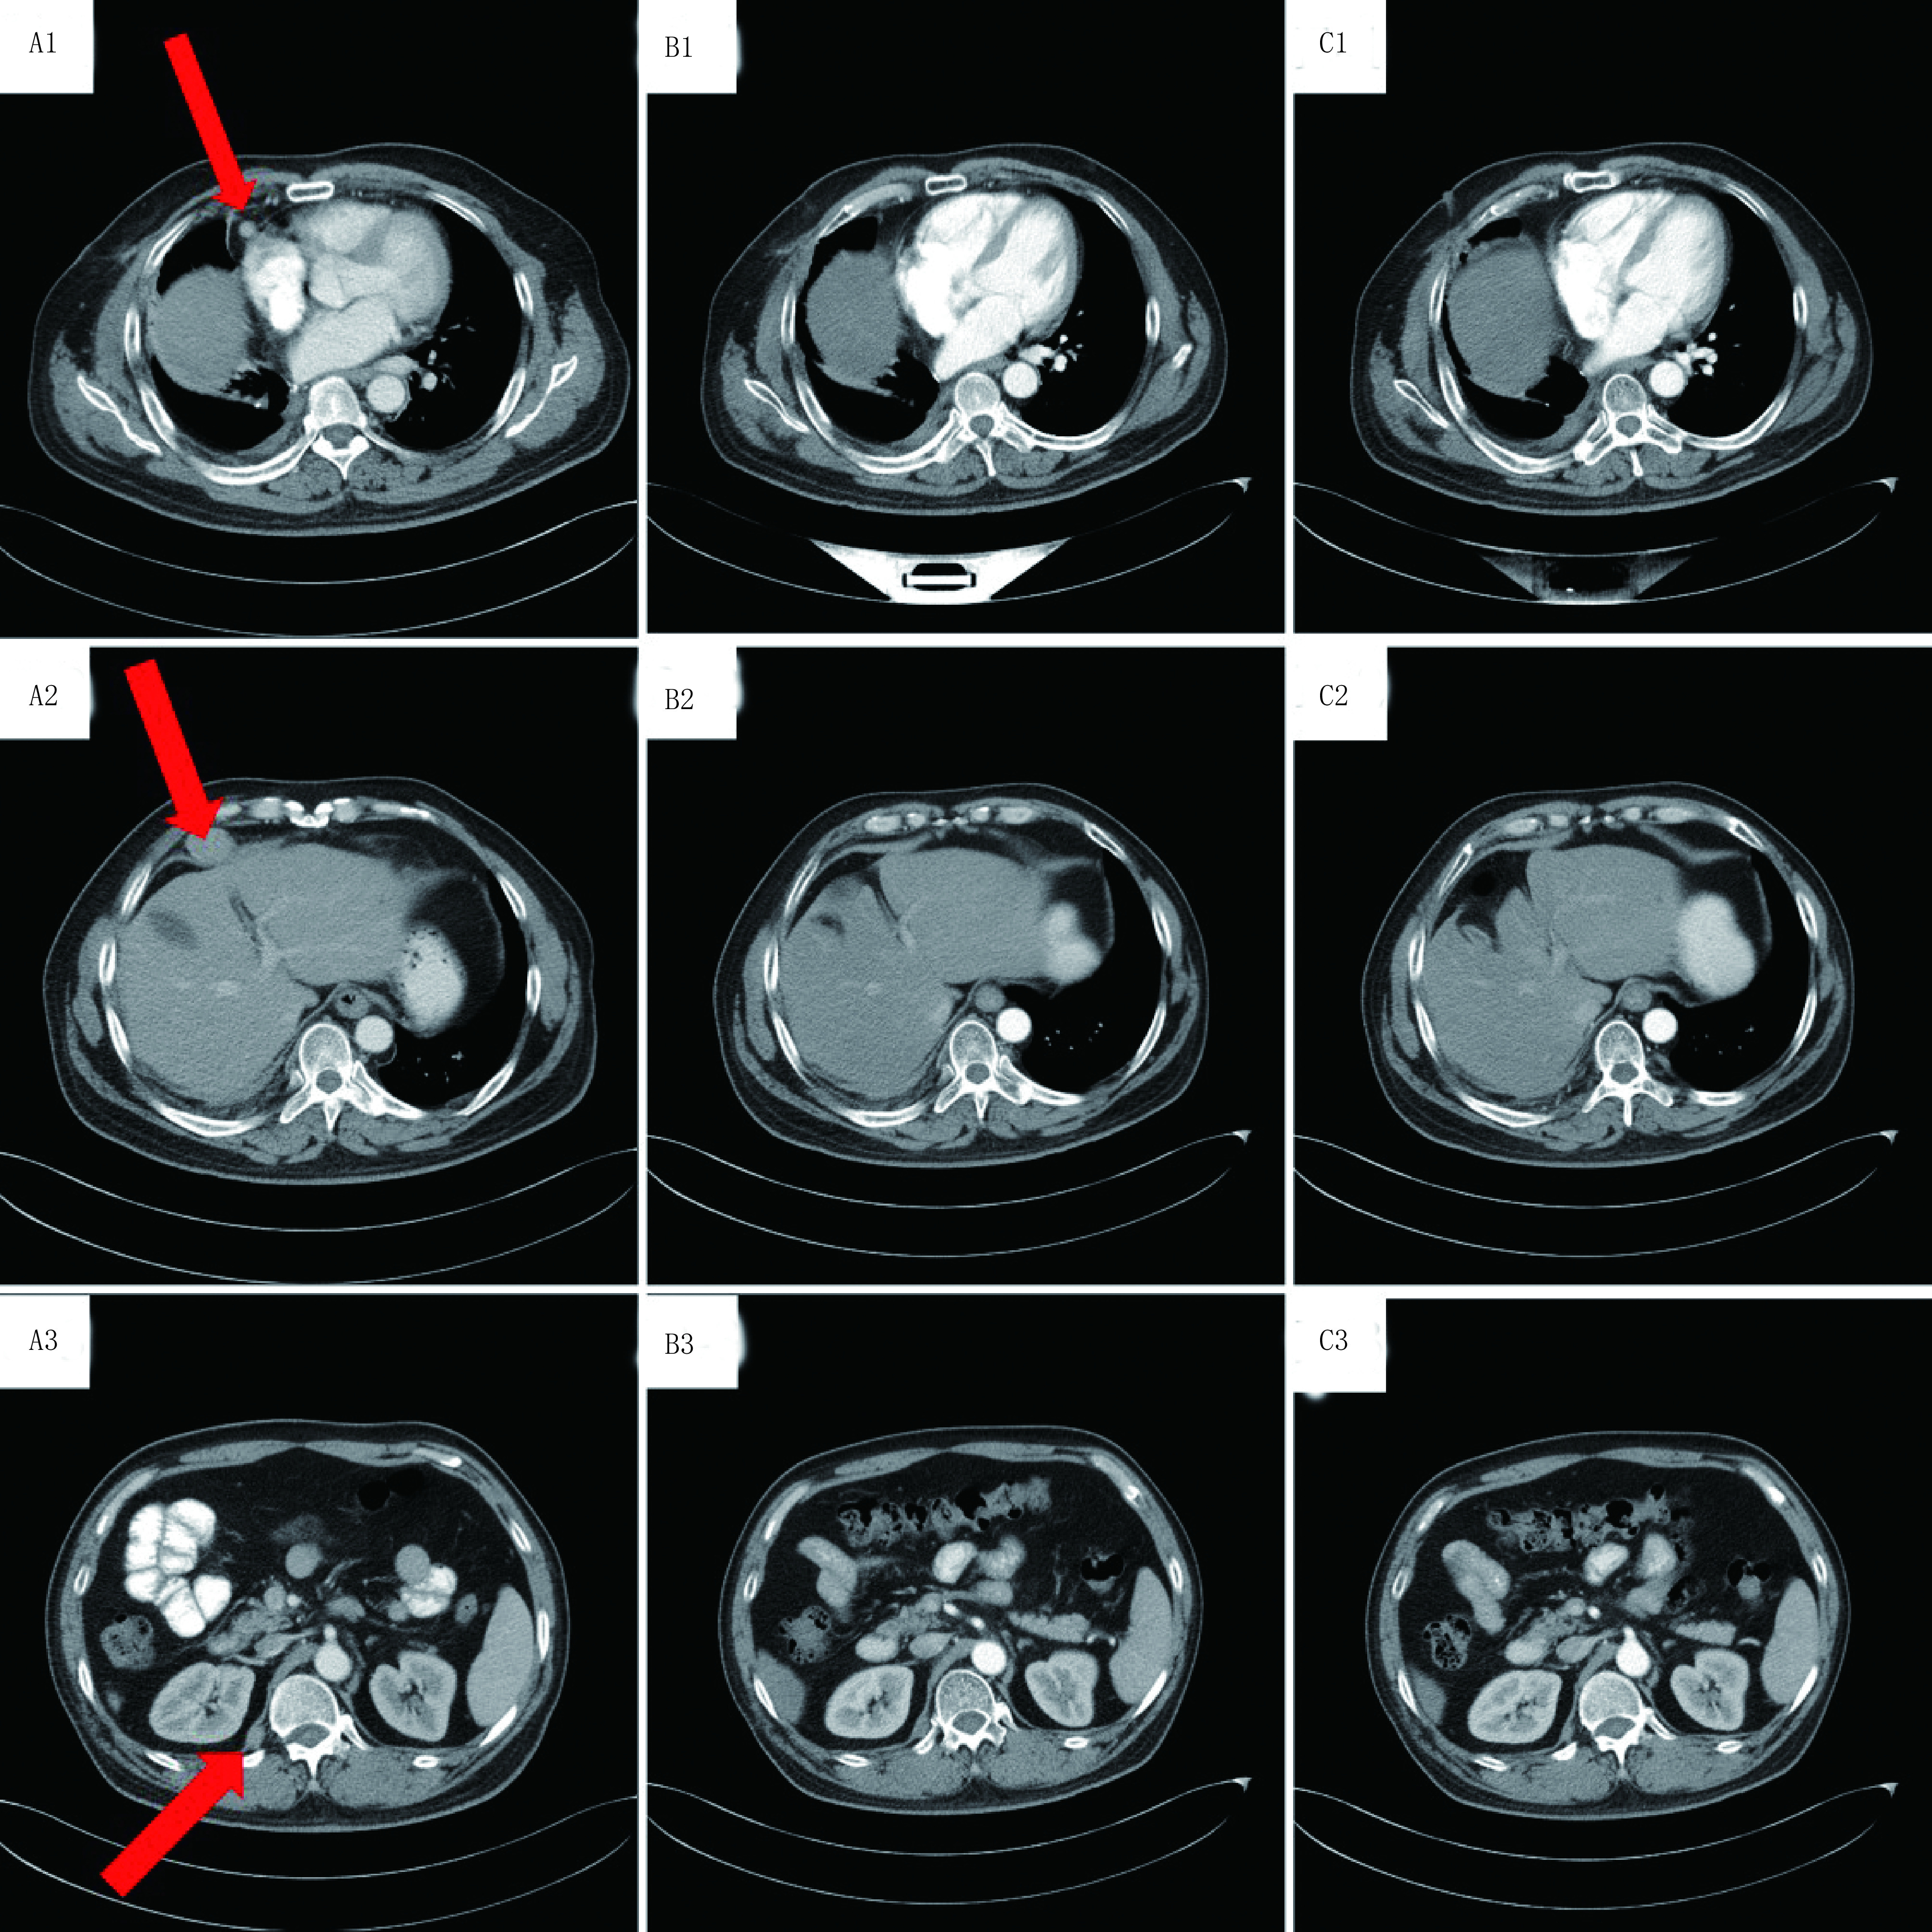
口服阿帕替尼治疗前后的CT扫描图像。阿帕替尼治疗前，2017年3月CT扫描显示右侧心膈角淋巴结转移、右侧椎旁结节灶转移（A1、A2、A3）。阿帕替尼治疗3个月后，2017年6月CT扫描显示转移灶均较前缩小（B1、B2、B3）。阿帕替尼治疗6个月后，2017年9月CT扫描显示病情稳定（C1、C2、C3）。 Chest CT scans before and after taking apatinib. March 2017 CT scans before apatinib therapy revealed metastasis of lymph node in cardiodiaphragmatic angle and right paravertebral (A1, A2, A3). After three months apatinib treatment, June 2017 CT scans showed that the metastatic nodules became smaller (B1, B2, B3). September 2017 CT images shows the condition was stable (C1, C2, C3).
